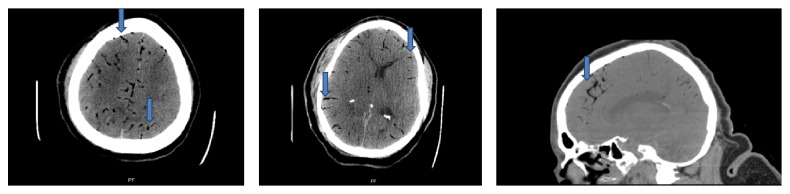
Case 2 CT scan of head: axial and sagittal cuts showing foci of gas in bilateral cerebral arteries (blue arrows).
